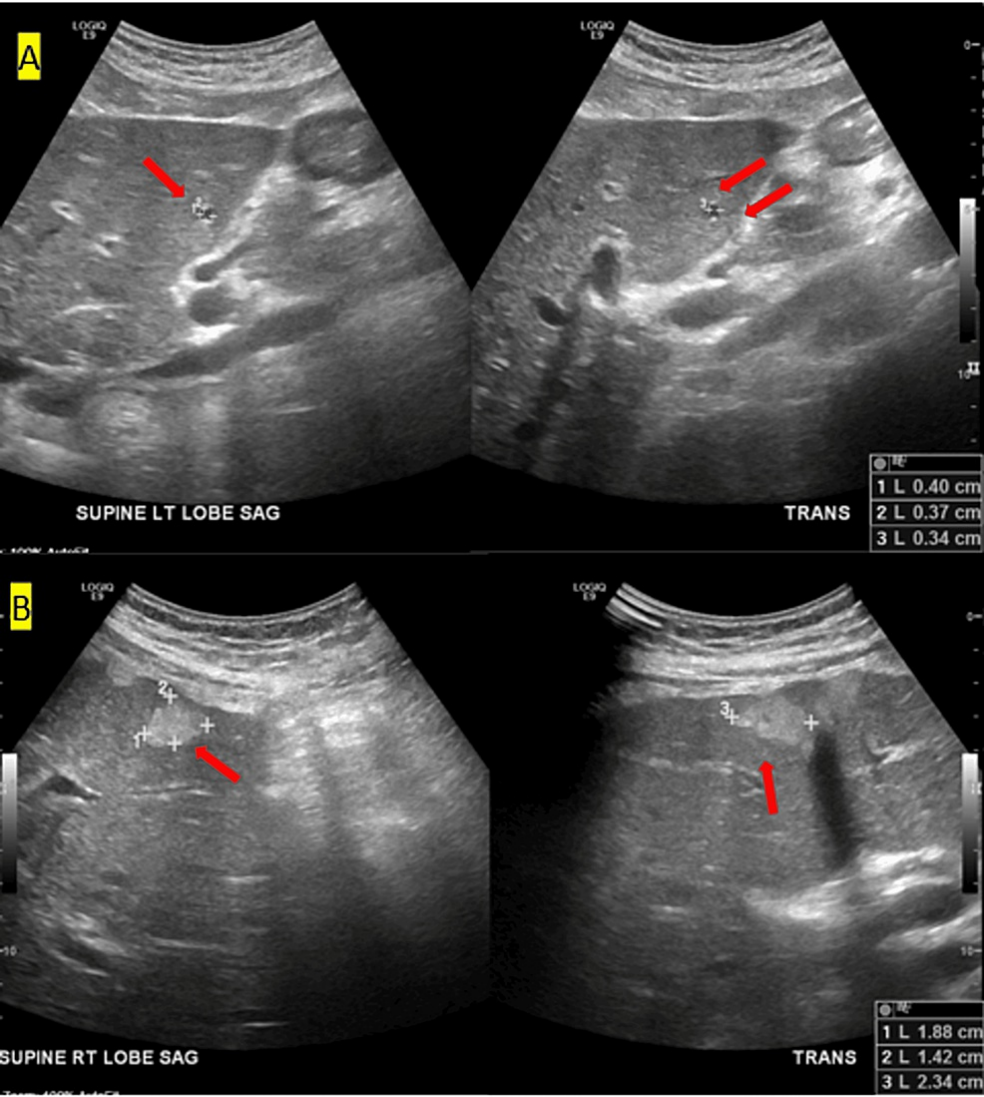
Initial liver ultrasound Figure A: Hepatomegaly with sub-centimeter hepatic cysts on the left lobe; Figure B: Hyperechoic lesions consistent with hemangiomas on the right lobe.
